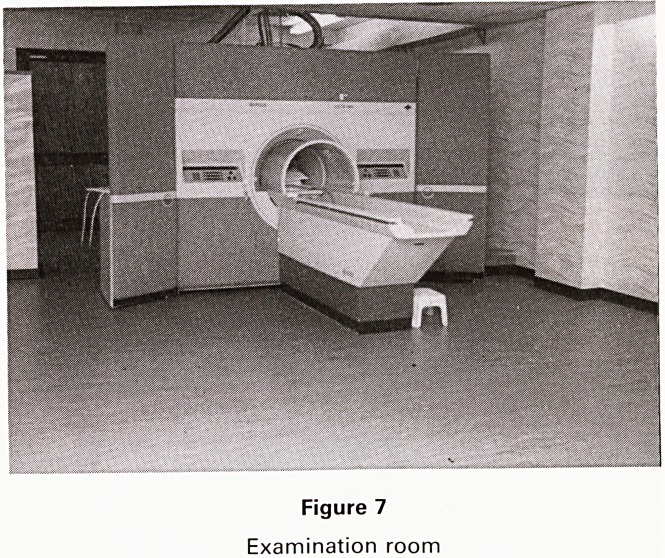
# The Bristol MRI Centre
